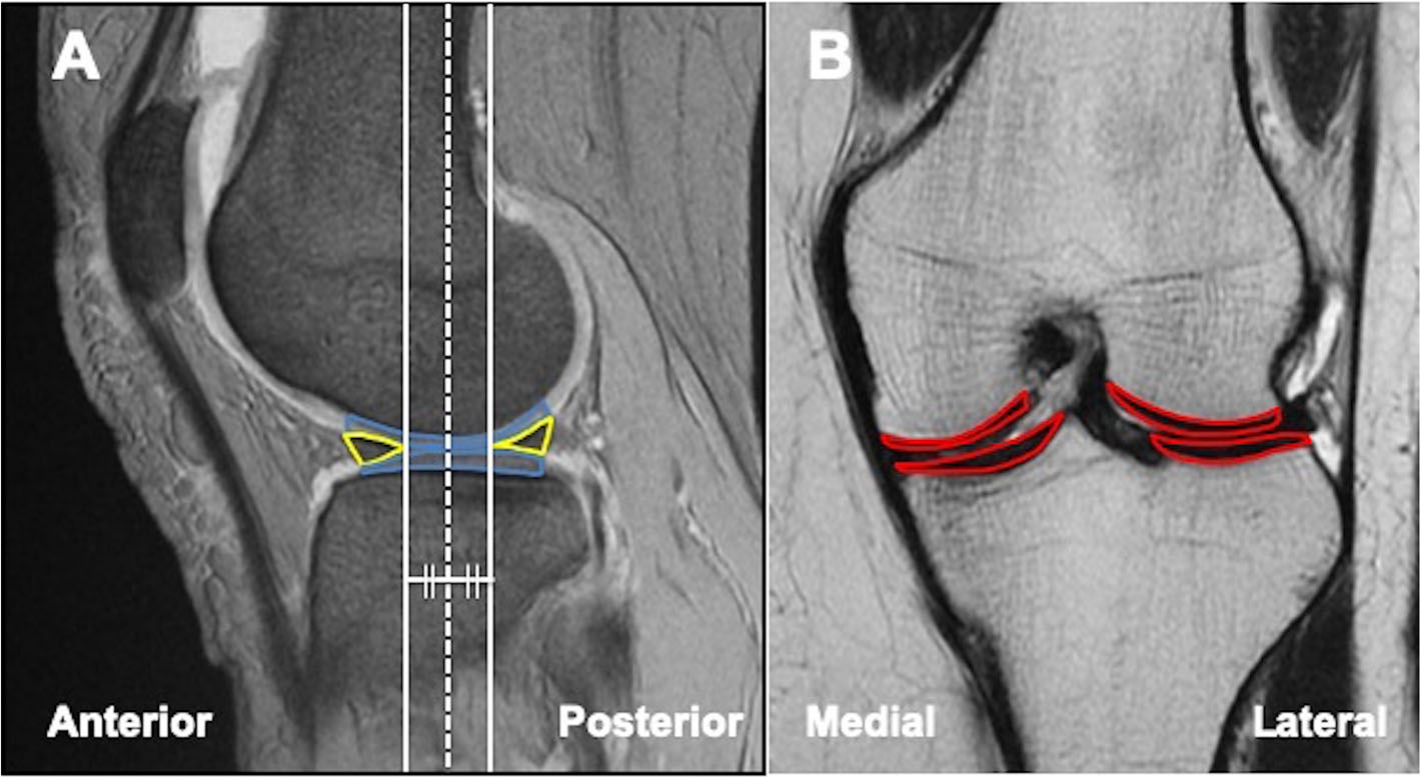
Method of calculating the T2 mapping value. a In a sagittal slice of T1-weighted fast-field echo images, we select a central slice (white dotted line) that passed through the center of the weightbearing cartilage (blue line) surrounded by the anterior and posterior margins of the meniscus (yellow line). In addition to the central slice, we added two slices neighboring the central slice anteriorly and posteriorly. b The region of interest (ROI) was set at the weight-bearing full-thickness cartilage (red line) of the medial and lateral femoral condyle and medial and lateral tibial plateau on the central slice of the coronal image. The ROI was also set using the same method on both the anterior and posterior slice. Overall, the T2 mapping values of 12 ROIs were measured
